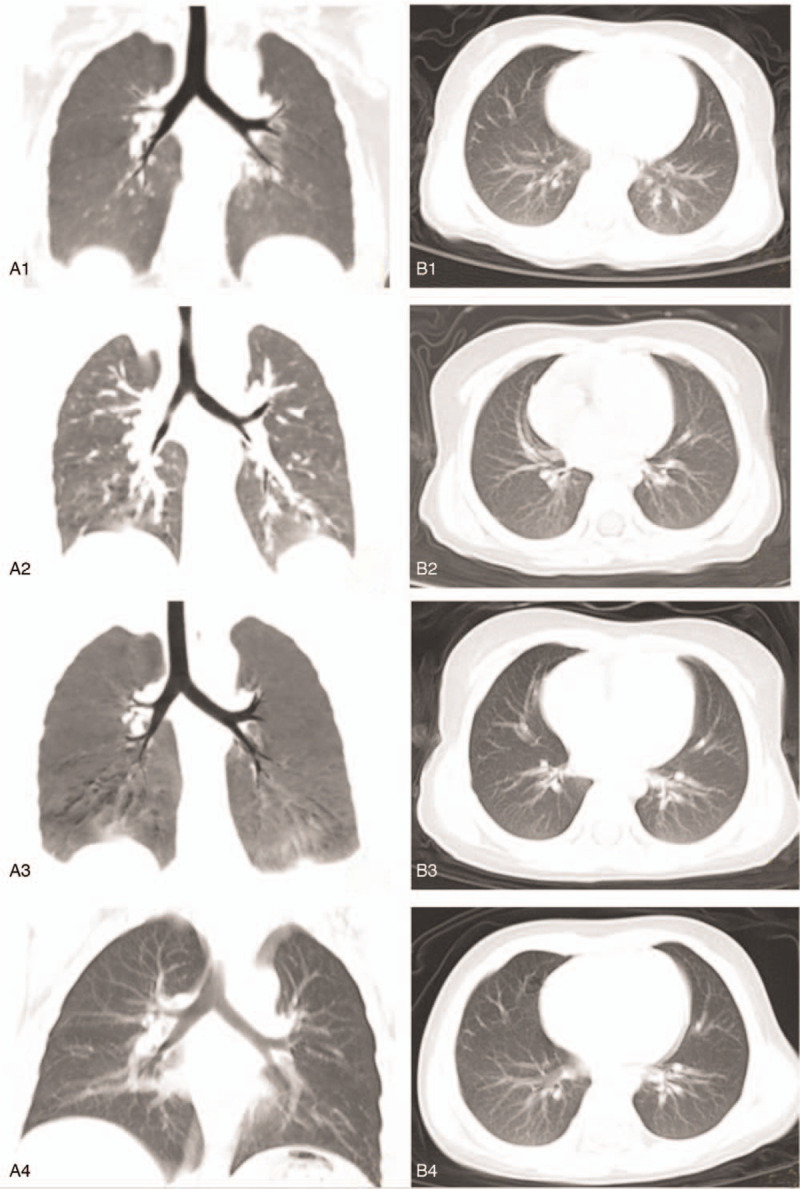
Chest CT. 1st day of admission (A_1_, B_1_). A_1_ Mediastinal window: Thymus shadow size and density are normal. The trachea and bronchi are unblocked. No displacement was observed in the mediastinum. B_1_ Lung window: Increased of lung markings. Small flaky fuzzy shadows can be seen in the right lower lobe of the lung. 19th day of admission (A_3_, B_3_). A_2_ Mediastinal window: As before. B_2_ Lung window: Fibrous stripes can be found in the upper lobe of right lung. The lesion in the lower lobe of the right lung has been absorbed. 4th day after discharge (A_3_, B_3_). A_3_ Mediastinal window: As before. B_3_ Lung window: The Fibrous stripes in the upper lobe of right lung just as before. 21th day after discharge (A_4_, B_4_). A_4_ Mediastinal window: As before. B_4_ Lung window: The Fibrous stripes in the upper lobe of right lung also as before. CT = computed tomography.
